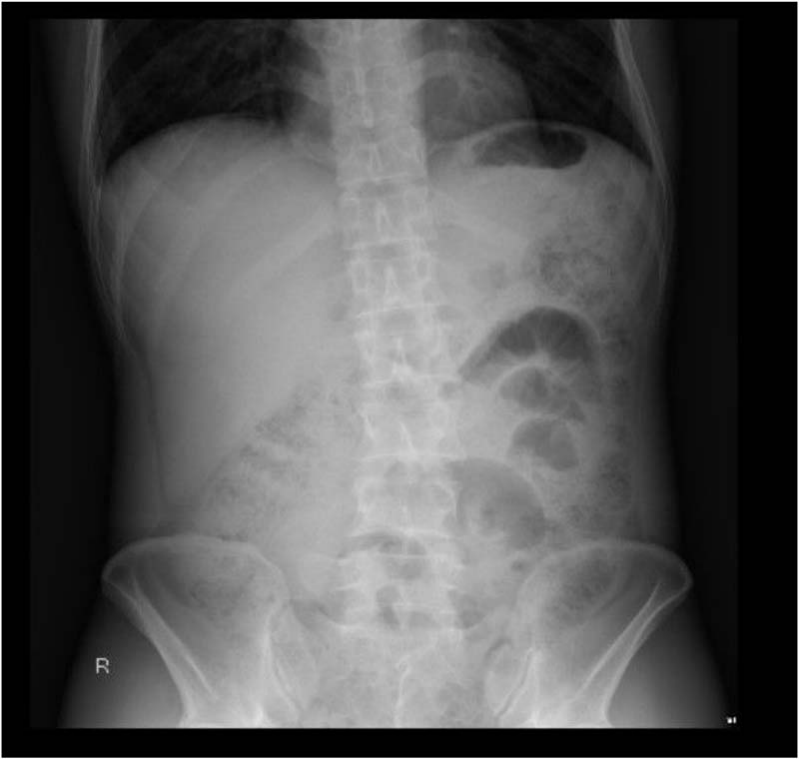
Abdominal X-ray with subtle evidence of pneumoperitoneum.
